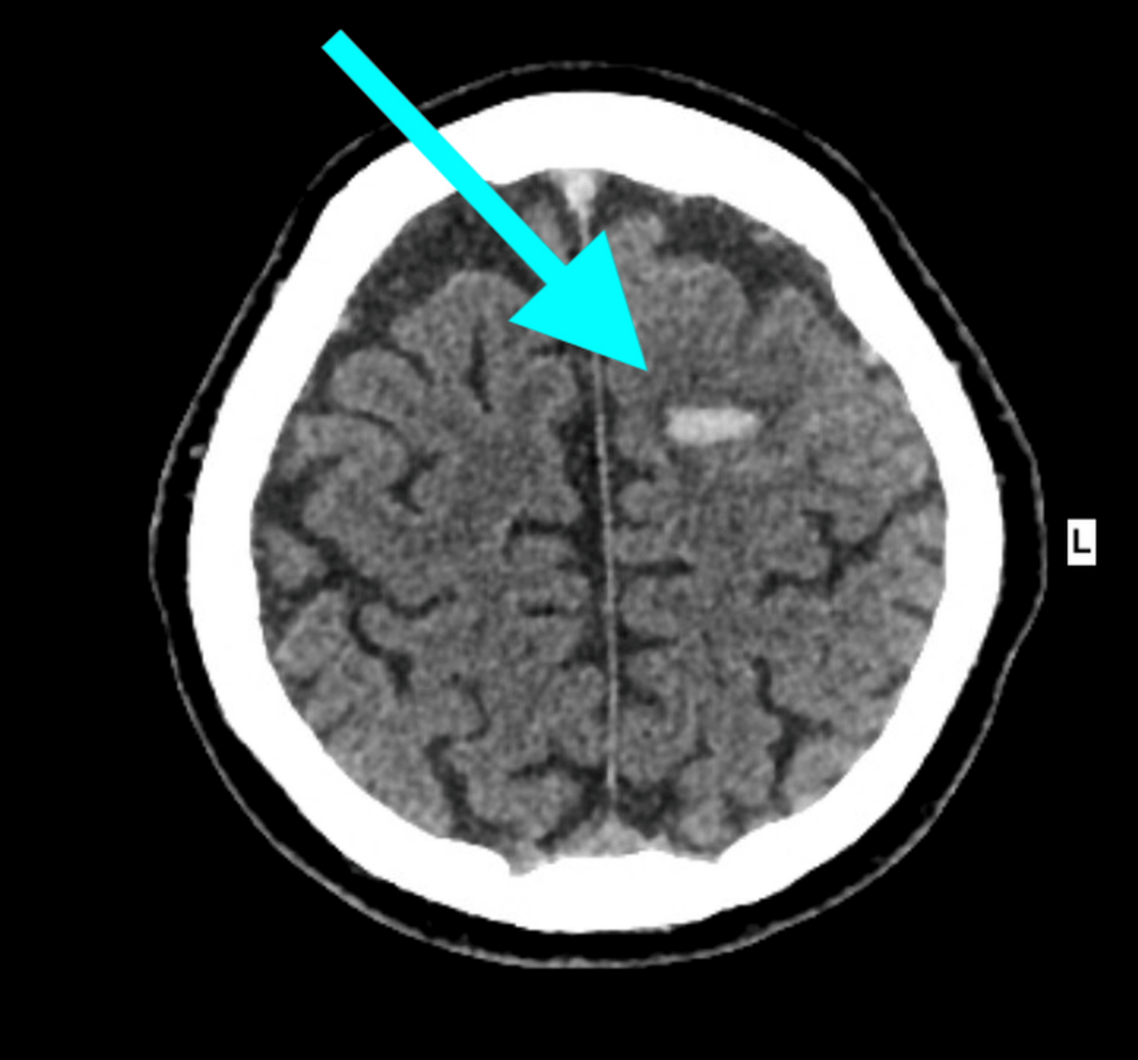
Non-contrast CT head shows a small acute intracerebral haemorrhage in the superior portion of the left frontal lobe (blue arrow)
